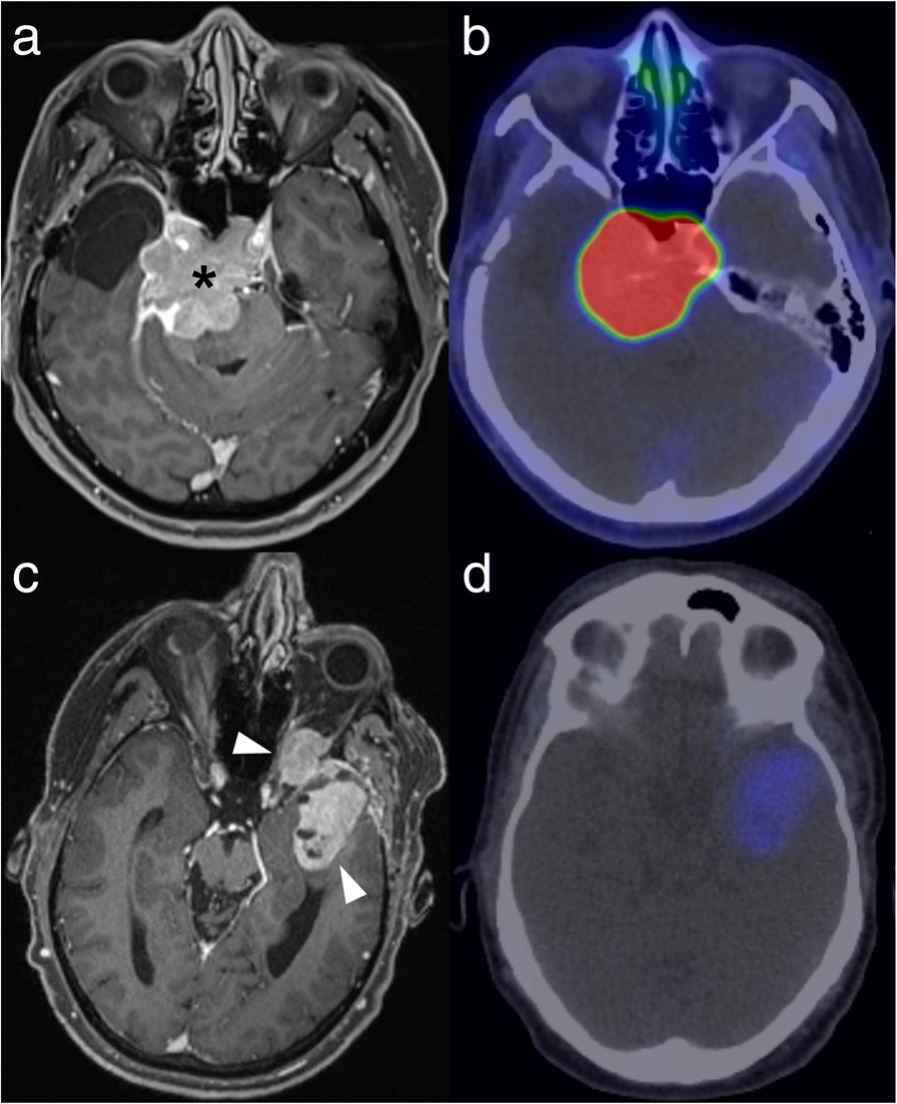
Post-contrast MRI (left) and GaTate-PET (right) images of two different patients with meningiomas refractory to conventional therapy. The skull base meningioma (a; asterisk) demonstrates high GaTate uptake (b) and may benefit from PRRT. In contrast, the left temporal meningioma extending into the orbit (c; arrowheads) has only low-grade GaTate uptake (d), thus PRRT is not warranted
